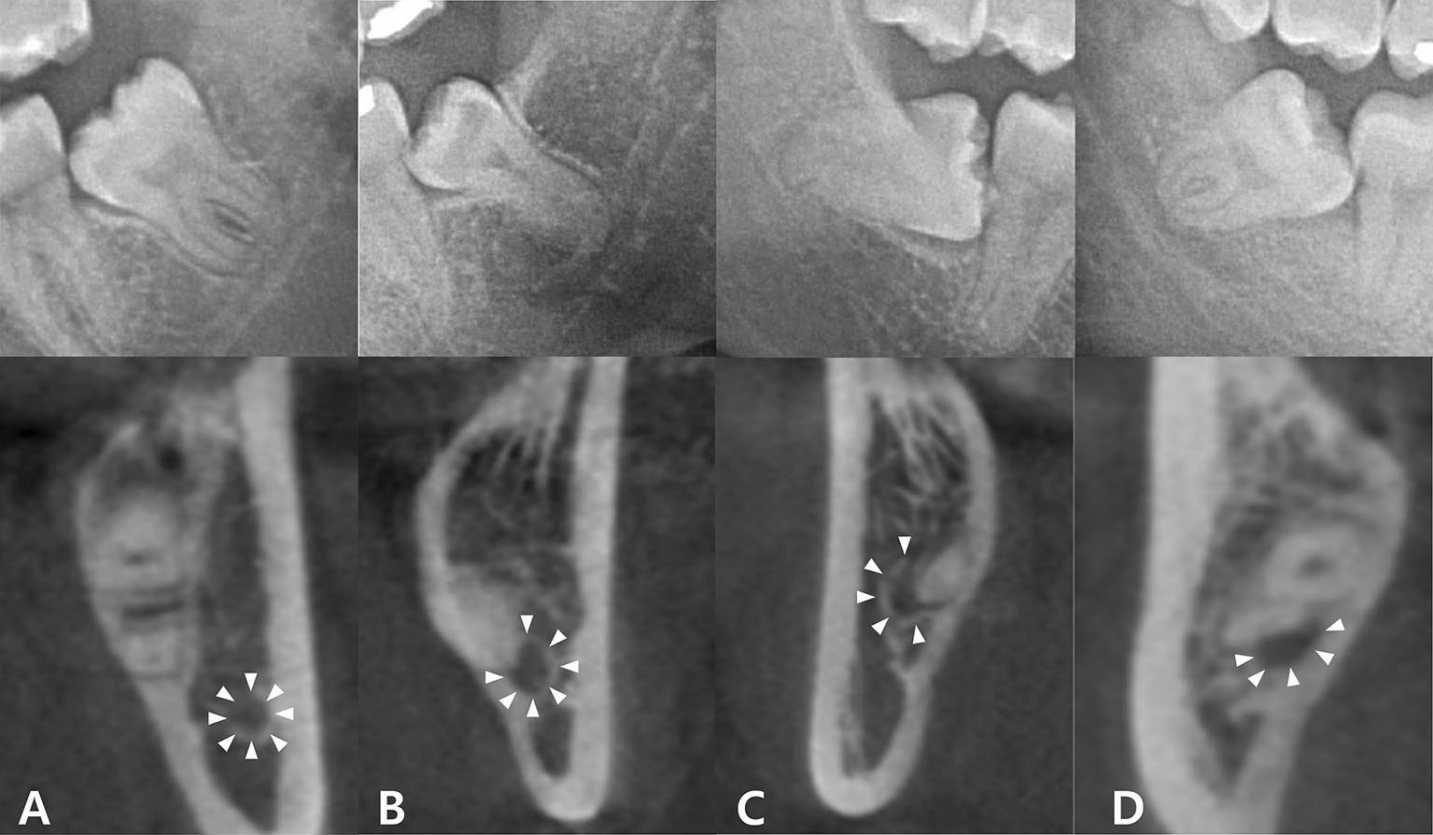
Classification of panoramic images based on CBCT. The M3 and IAN seemed to be superimposed in four panoramic images. White triangles point to the border of the IAN in CBCT. (A) Intimate but non-contact positioning between M3 and IAN. (B) True contact positioning between M3 and IAN. (C) IAN positioned buccal to M3. (D) IAN positioned lingual to M3. *CBCT* cone-beam computerized tomography, *M3* mandibular third molar, *IAN* inferior alveolar nerve.
